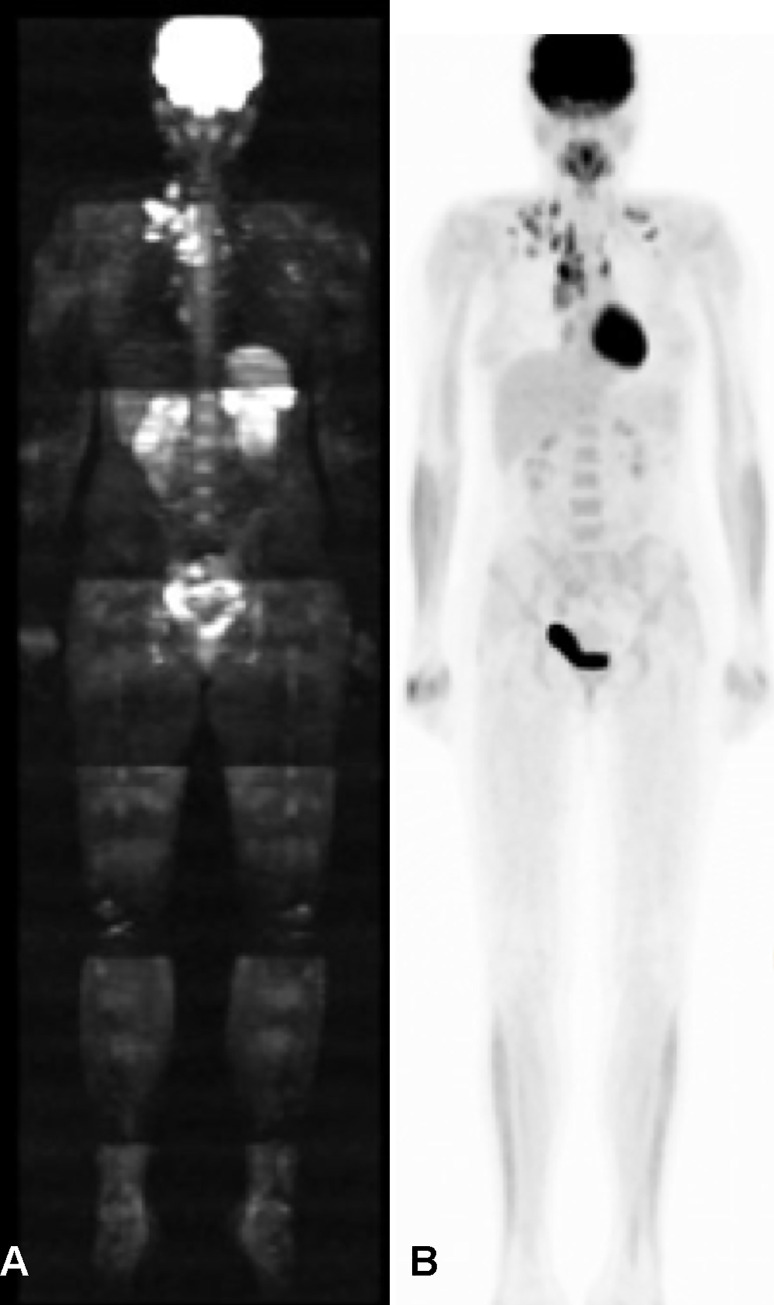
Female patient, fifteen-year-old, with Hodgkin's lymphoma, nodular sclerosis type. These images in the coronal plane, (A) diffusion and (B) PET-CT, show mediastinal and supraclavicular lymph node enlargement.
